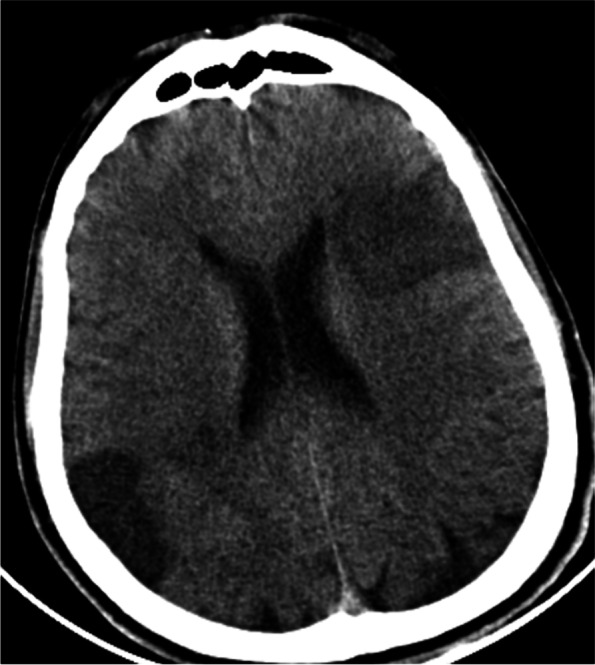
Brain CT scan 24 h after admission showed a new infarct in the left frontal lobe
